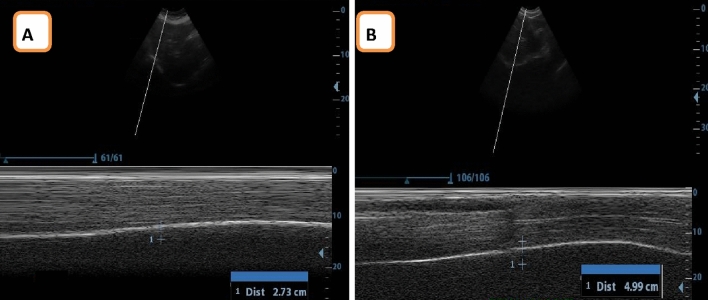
Ultrasound measurement of Diaphragmatic excursion (A) VL group, (B) control group.
